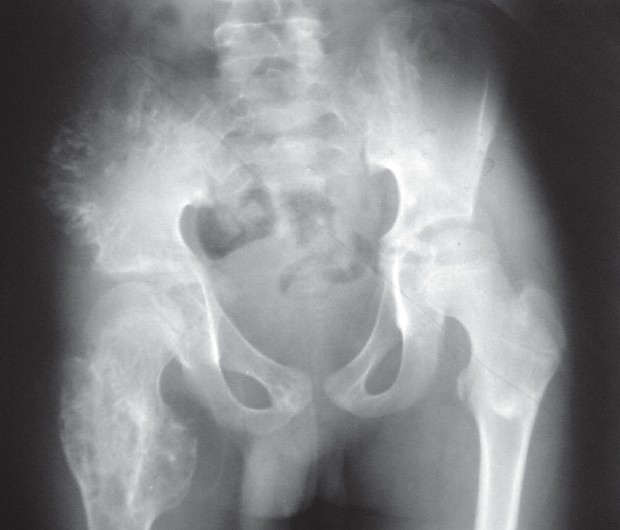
Anteroposterior radiograph of the pelvis showing columnar, radiolucent lesions in the right ilium extending to the crest and suggestive of cartilaginous rests. Similar lesions are seen in the greater trochanter of the right femur.
